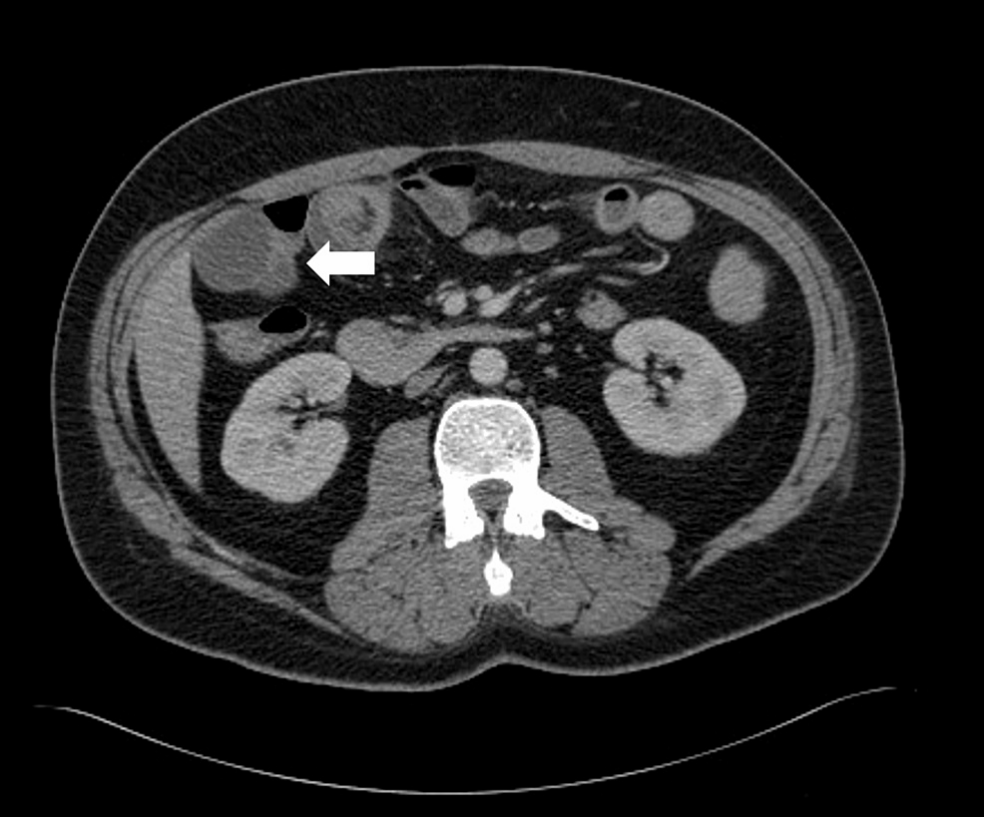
Axial contrast-enhanced CT image obtained at presentation with abdominal pain, showing evidence of target-like appearance in the subhepatic short segment of the small bowel measuring about 2.7 cm indicating small bowel intussusception.
